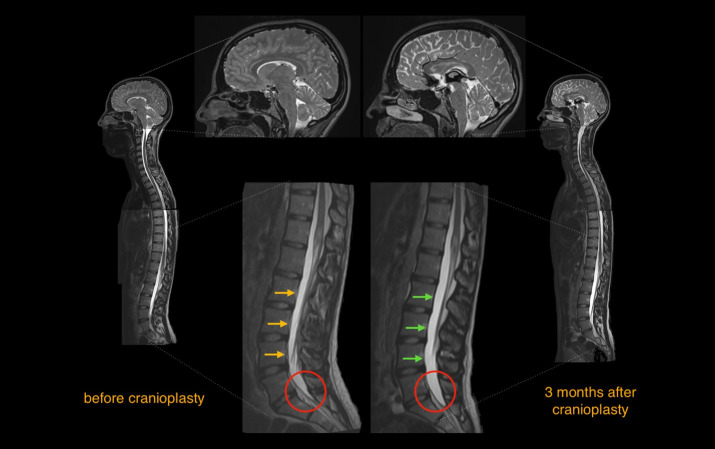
Sagittal whole-neuroaxis magnetic resonance imaging (MRI) obtained before cranioplasty (left) and 3 months after cranioplasty (right) using an identical protocol. Insets, upper row: enlarged sagittal head MRI clearly shows reduced subarachnoid spaces with cranial defects (left) and their restoration upon cranioplasty; lower row: enlarged lumbosacral sagittal MRI – reduced cerebrospinal fluid (CSF) volume provoked the replacement of a retrovertebral area with fatty tissue (yellow arrows) and refilling of the same spaces with CSF after cranioplasty (green arrows). Red circles show marked differences in the fullness of the terminal dural sac in respect to cranial defect vs restoration of cranial integrity.
